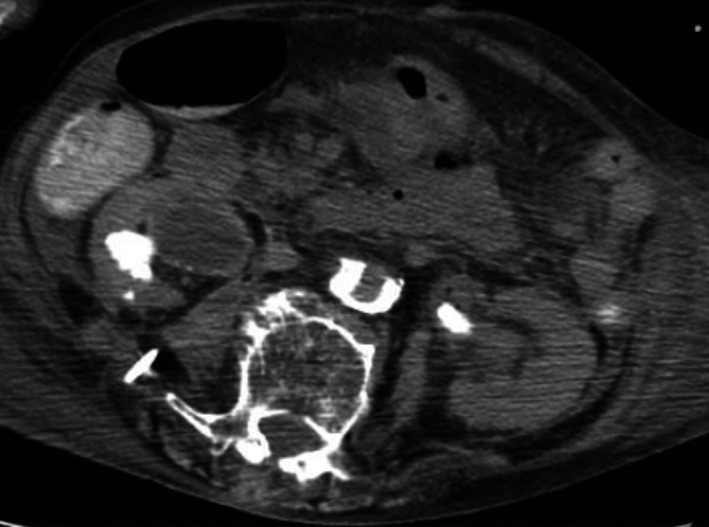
A noncontrast abdominal computed tomography (CT). An abdominal noncontrast CT revealed a marked thinning of the right renal cortex and large staghorn calculi filling the middle calyces in both kidneys
